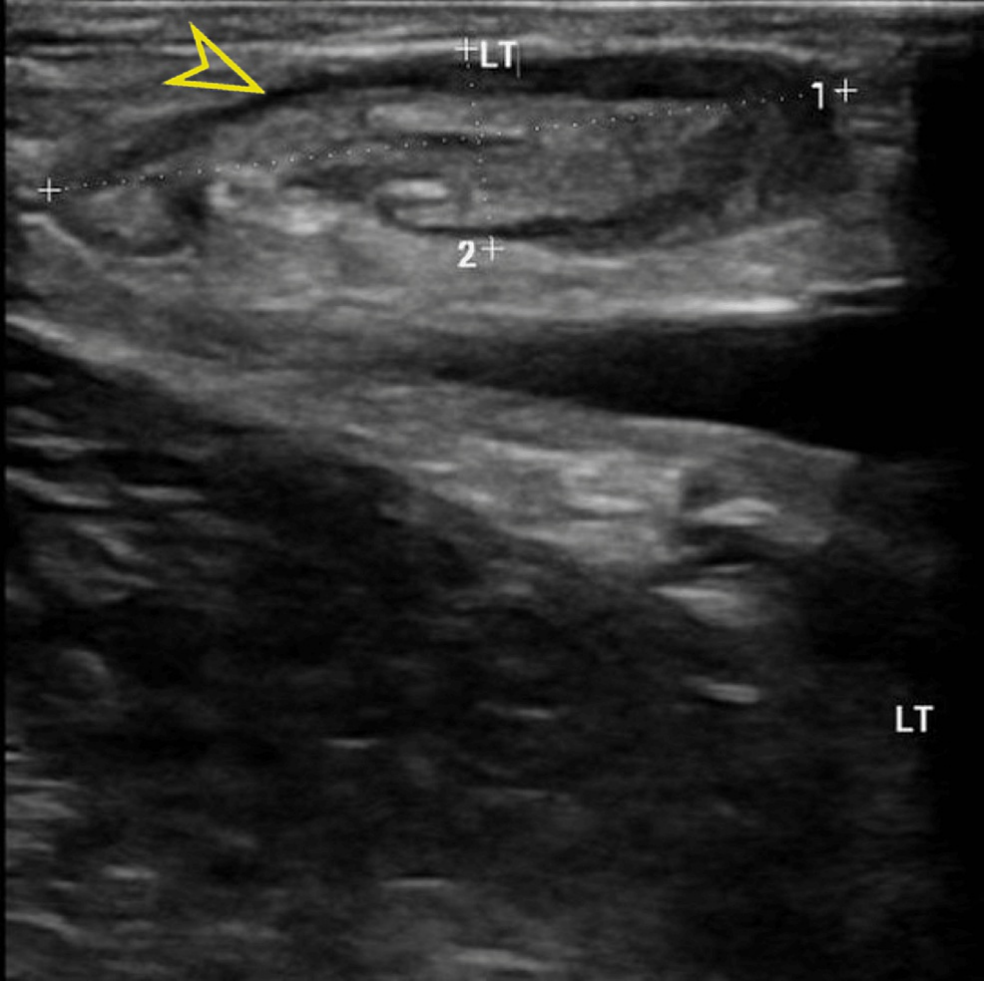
Correlated Inguinal ultrasound shows multiple prominent bilateral oval-shaped inguinal lymph nodes with preserved fatty hilum.
